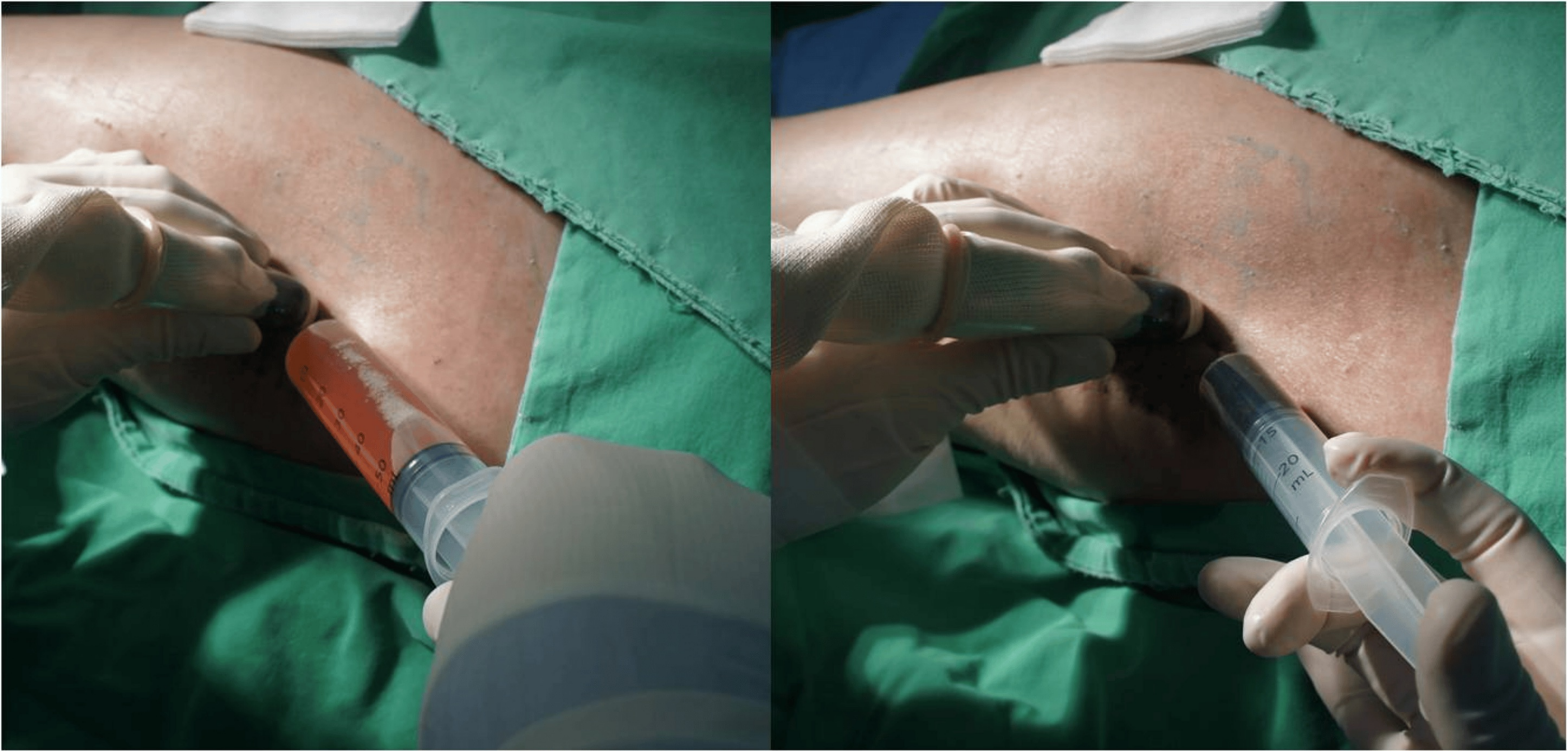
Ultrasound-guided sclerotherapy for recurrent Morel-Lavallée lesion Ultrasound-guided aspiration was performed at four weeks postoperatively, yielding approximately 200 mL of serous fluid (left). Subsequently, the prepared sclerosant solution (povidone-iodine, lidocaine, and saline mixture) was injected through the same needle and retained within the cavity without reaspiration (right), aiming to induce a fibrosing inflammatory response and obliterate the dead space.
